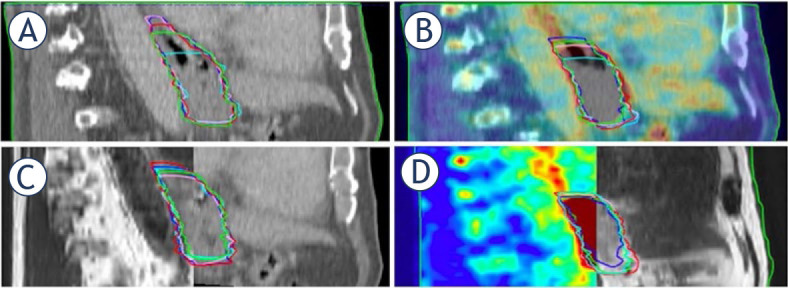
Delineation of the gross tumour volume (GTV) of all five observers of case 22, sagittal view. The variation in cranial border is highest on computed tomography (CT) and lowest on positron emission tomography (PET/CT) magnetic resonance (MR). (A) CT; (B) PET/CT; (C) fusion of CT and MR; (D) fusion of PET/CT and MR.
